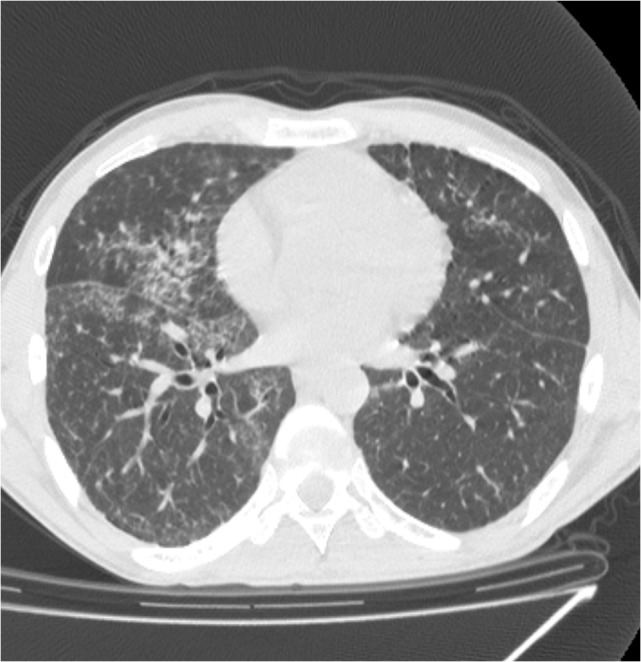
8 months follow-up CT-scan control after bilateral whole lung lavag
